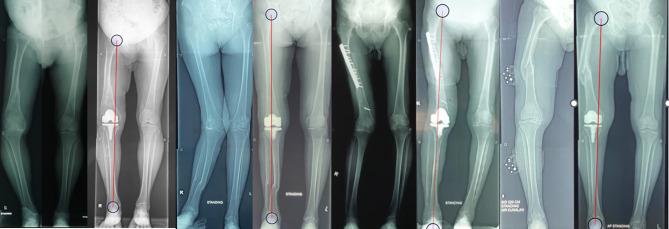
Preoperative Scannogram and Postoperative Scannogram (Radiograph showing long limb Anterior-Posterior view of four patients with red colour line corresponding to lower limb axis from center of hip joint to center of ankle joint).
